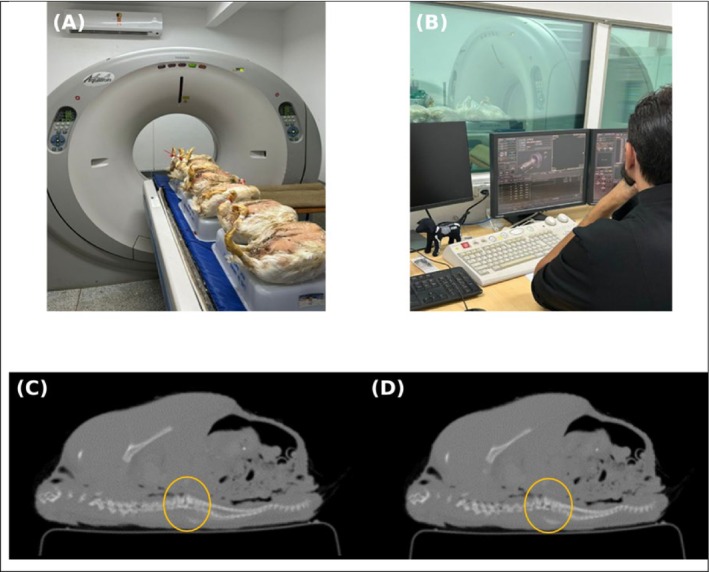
Evaluation of spondylolisthesis using CT scan (A), image of the CT scan procedure (B). Evaluation of spondylolisthesis with an image obtained from a CT scan, demonstrating the spinal cord. Letters C and D indicate the lesion.
